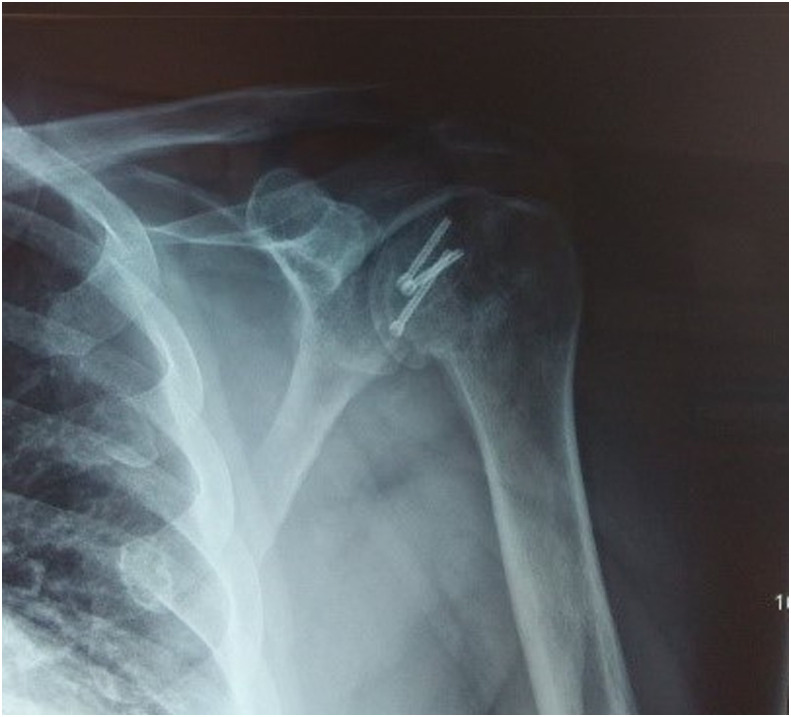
2nd patient: Postoperative (AP) radiographs of Left shoulder. Metal screws were used for lesser tubercle transfer-fixation.
